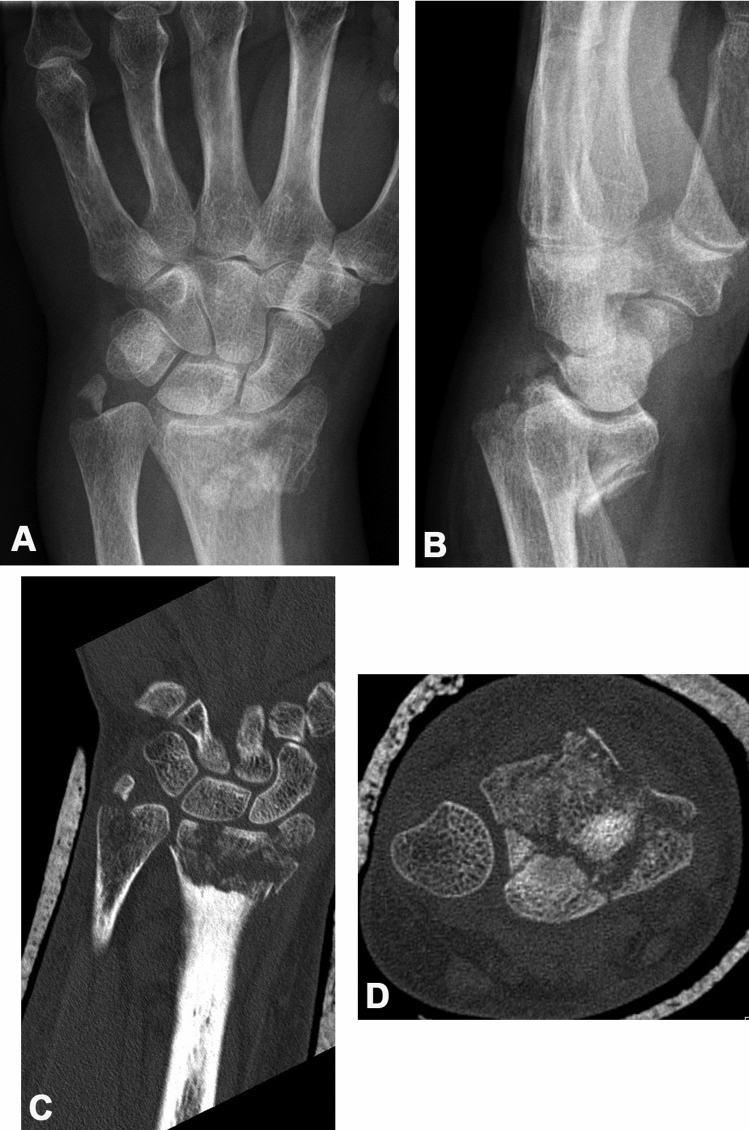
Distal radius fracture in a 61-year-old female patient. Using standard posteroanterior (a) and lateral (b) radiographs, this fracture was classified as a type IV fracture according to the Frykman classification. The coronal (c) and axial (d) CT reconstructions, however, clearly show fracture extension into the sigmoid notch
